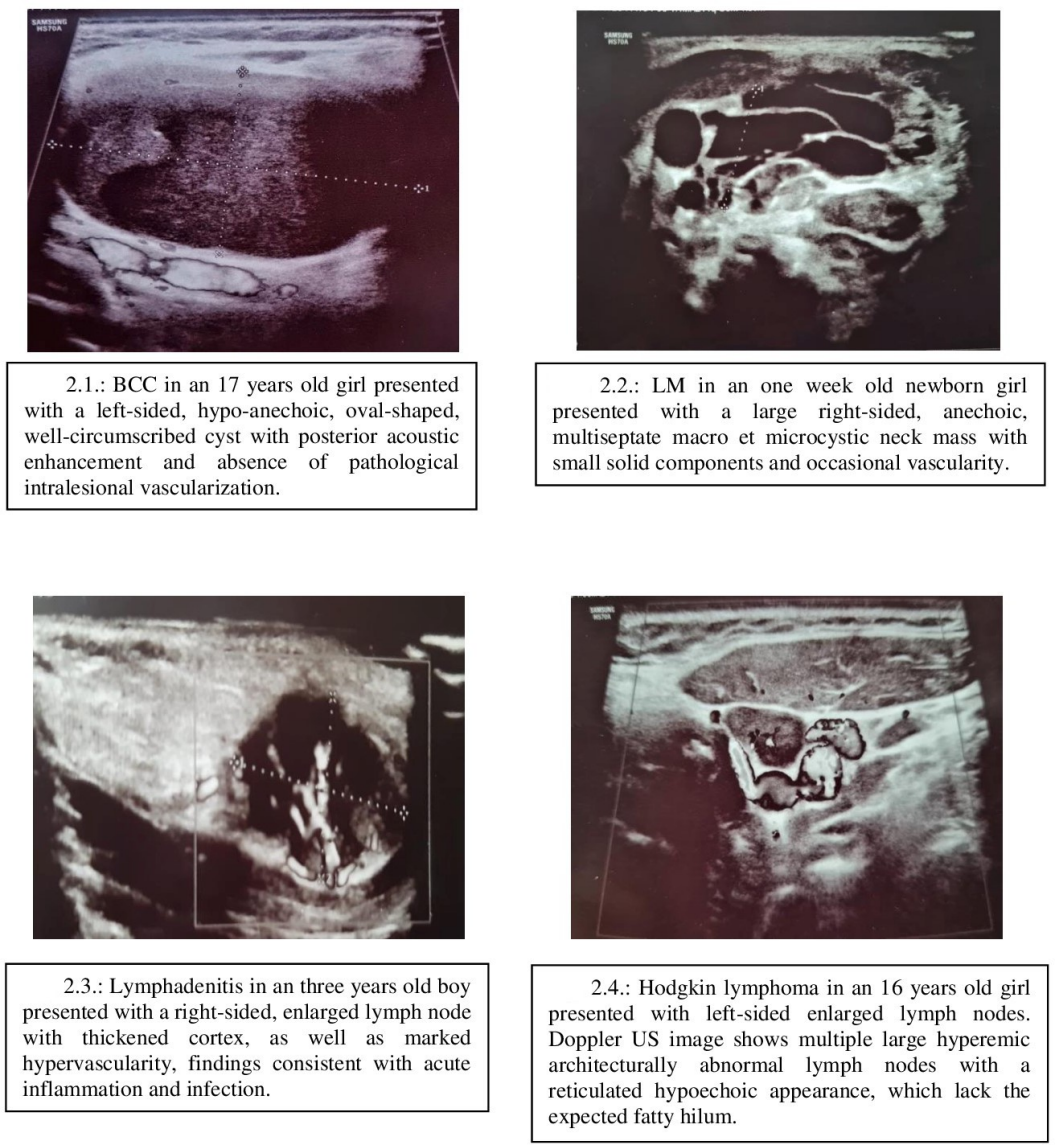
Images of lateral neck masses ultrasound findings (BCC-branchial cleft cyst; LM-lymphatic malformations; US-ultrasound).
